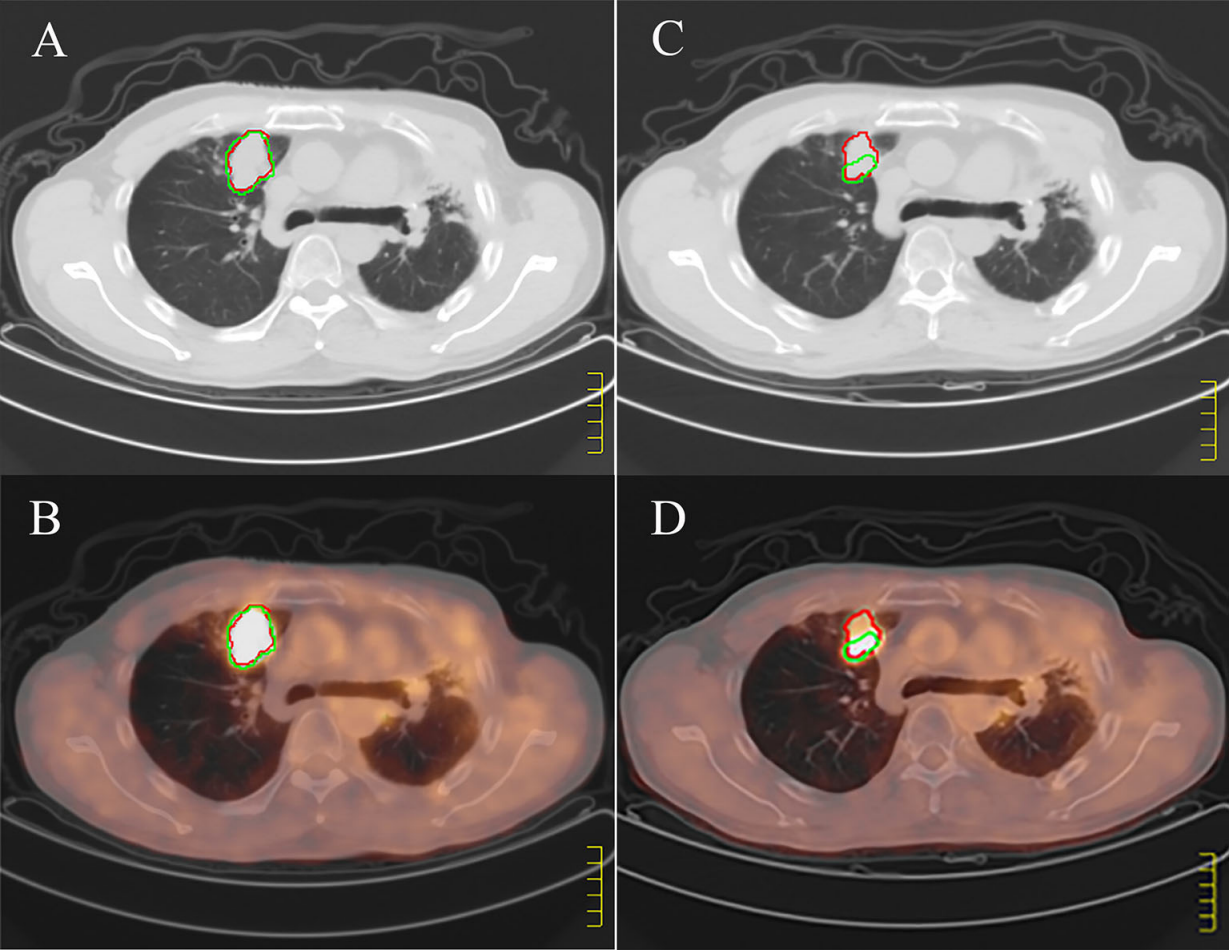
Example of discordant positron emission tomography (PET/CT) and computed tomography (CT). (A, B) show pretreatment CT and PET/CT images respectively, and (C, D) show CT and PET/CT images of the large tumors 3 months after treatment. The red regions of interest (ROI) is manually segmented based on CT, and the green ROI is delineated according to SUV value higher than 2.5 based on PET. According to the images before and after treatment, CT shows stable disease; PET/CT shows partial response.
